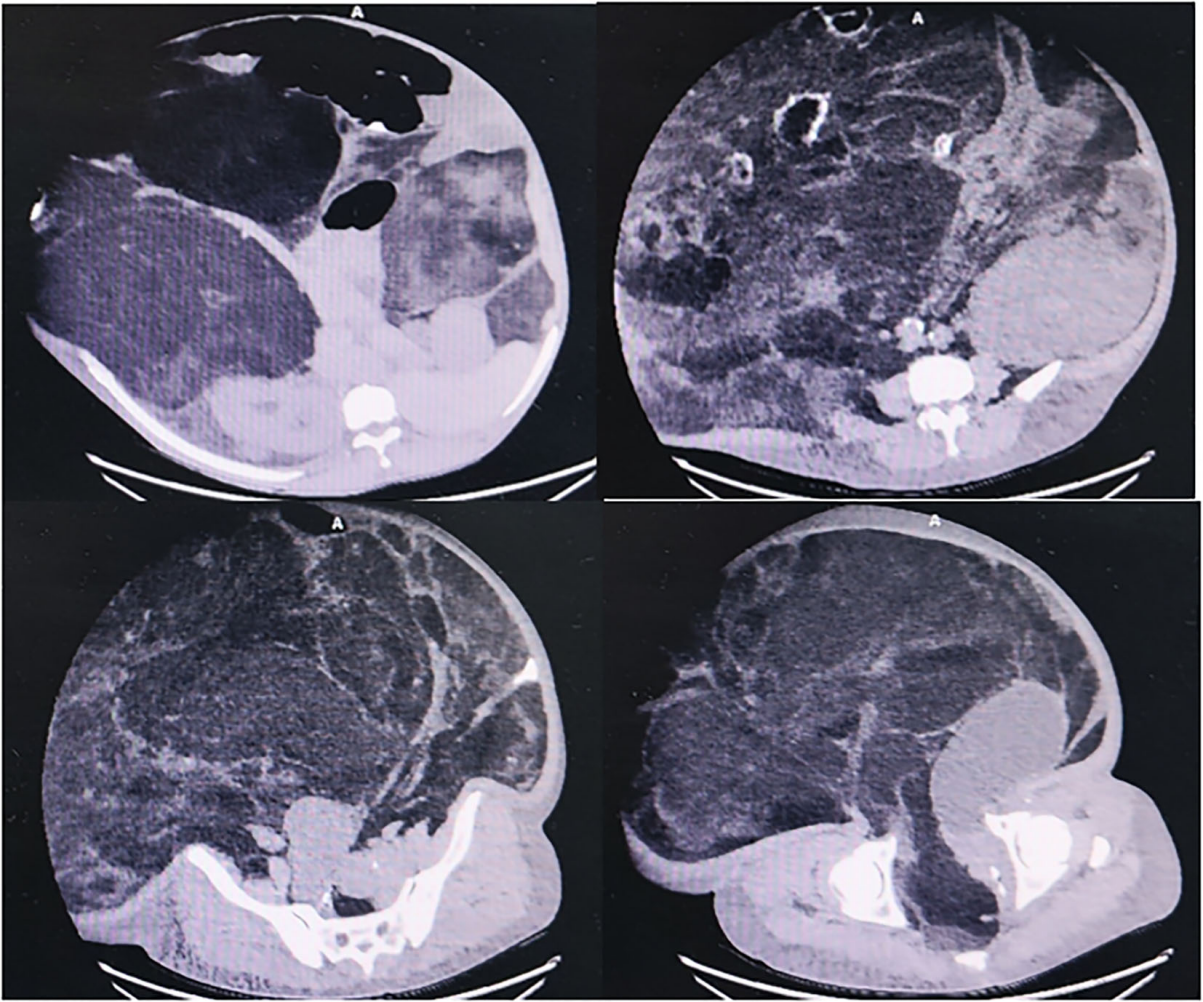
The patient’s CT images.
